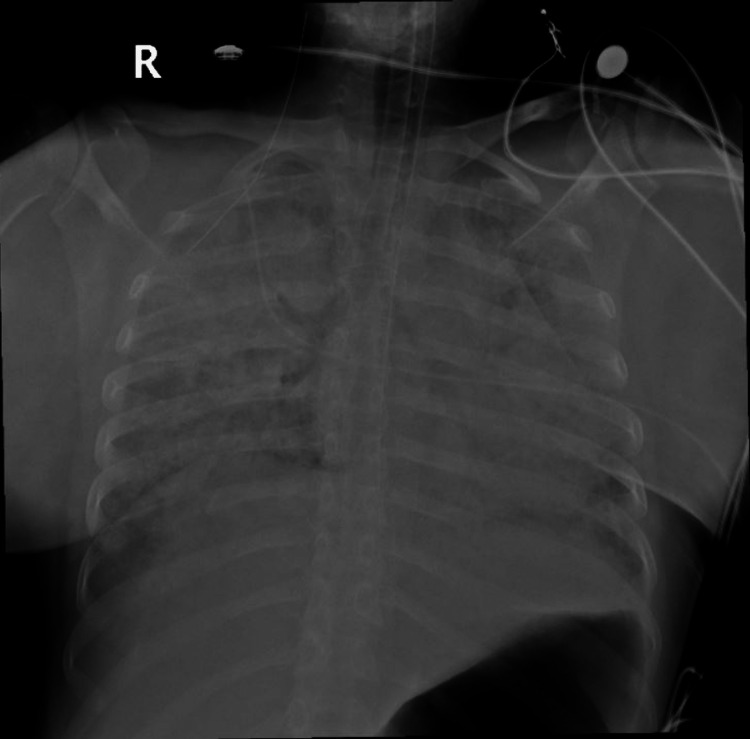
Chest x-ray (anteroposterior view) after intubation
